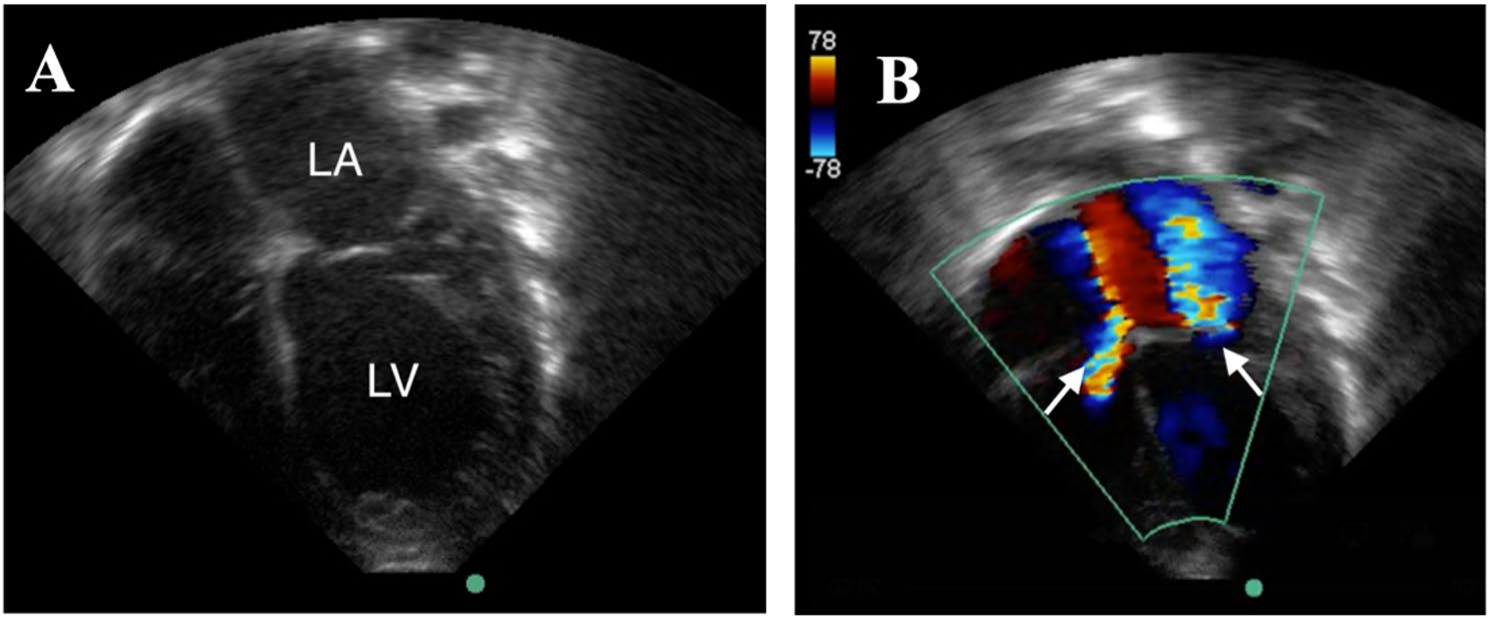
Transthoracic echocardiography demonstrating apical four-chamber view. A Apical four-chamber view demonstrating severe left atrial and left ventricular dilation. LA – left atrium, LV – left ventricle. B Apical four-chamber view demonstrating severe mitral and tricuspid regurgitation (arrows)
